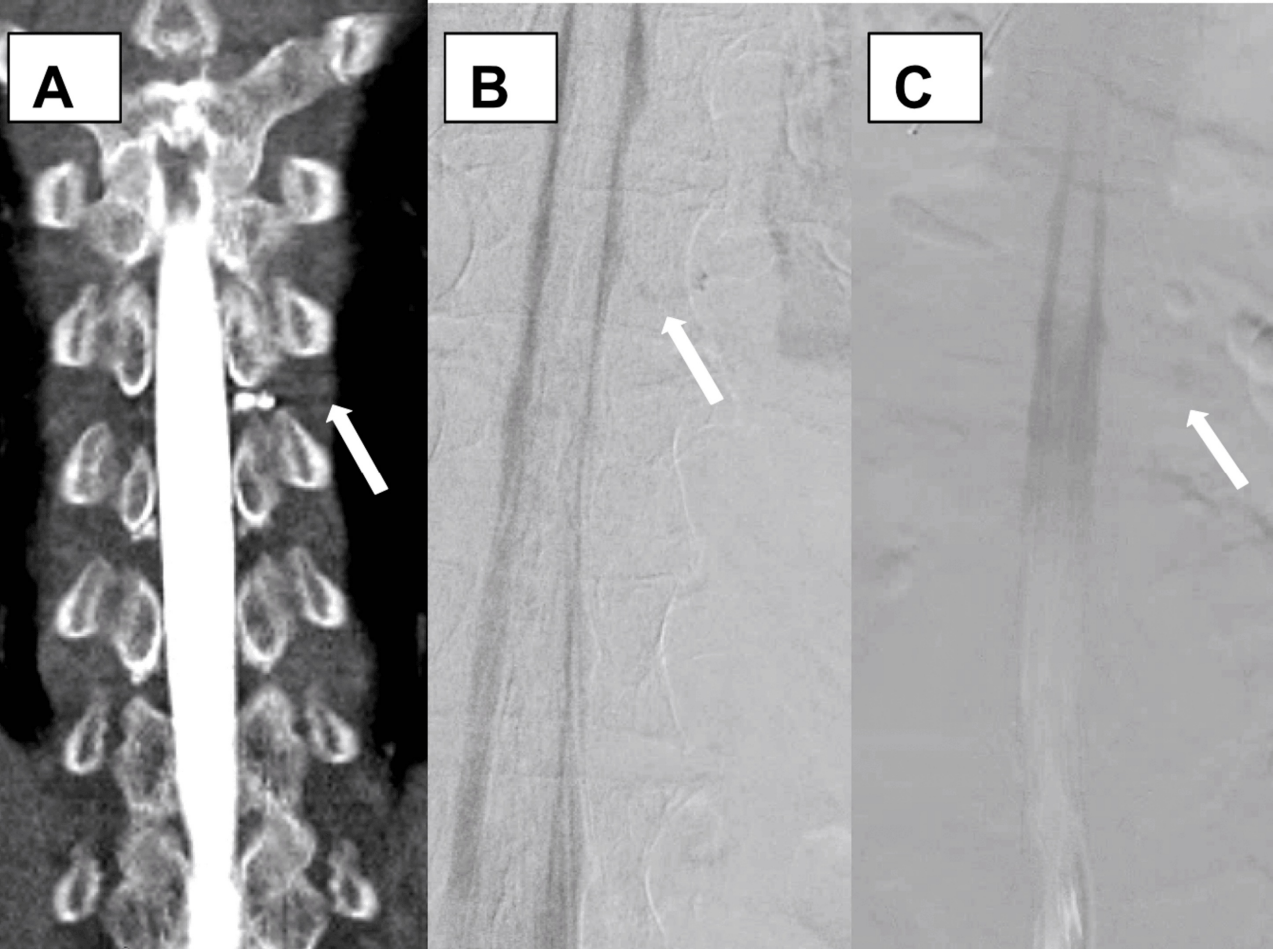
CT Myelography Spine A: Supine CT myelography reveals abnormal signal near left T7 nerve root (arrow) B: Prone digital subtraction myelography reveals CSF-venous fistulas near right T7 nerve root (arrow) C: Prone digital subtraction myelography reveals CSF-venous fistulas near right T10 nerve root (arrow) CT - Computerized Tomography T7 - Thoracic level 7 CSF - Cerebrospinal Fluid T10 - Thoracic level 10
